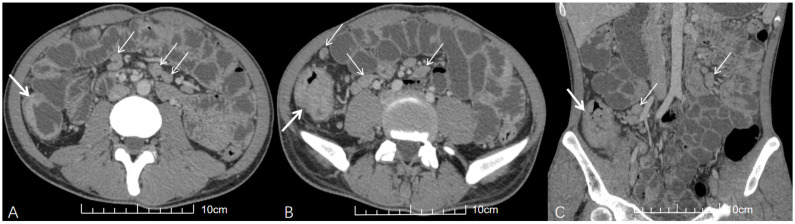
A young HIV-negative patient with abdominal pain and diarrhea for 1 month. (A,B) Axial and (C) coronal contrast-enhanced abdominal CT (venous phase) images demonstrate multiple enlarged lymph nodes in the mesentery with homogeneous enhancement and no fusion (thin white arrows). Concurrent ileocecal intestinal TB is evidenced by circumferential wall thickening (thick white arrows in (A–C)).
